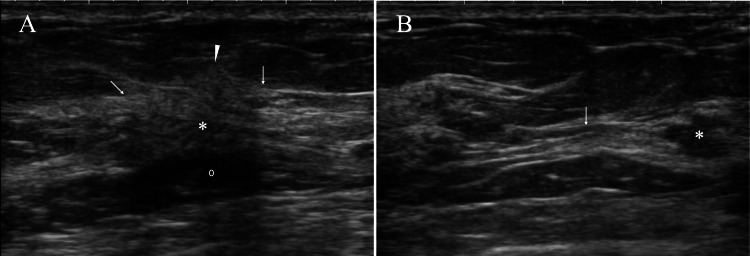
Ultrasound findings (A) Ultrasound showed an indistinct mass (asterisk), disruption of the anterior borders of the mammary gland (arrows), hyperechoic areas just above the mammary gland (arrowhead), and slightly attenuated posterior echoes (open circle). (B) Ultrasound showed a daughter nodule (asterisk) in the nipple direction of the main tumor and tubular low echoes (arrow) extending from the daughter nodule toward the nipple.
